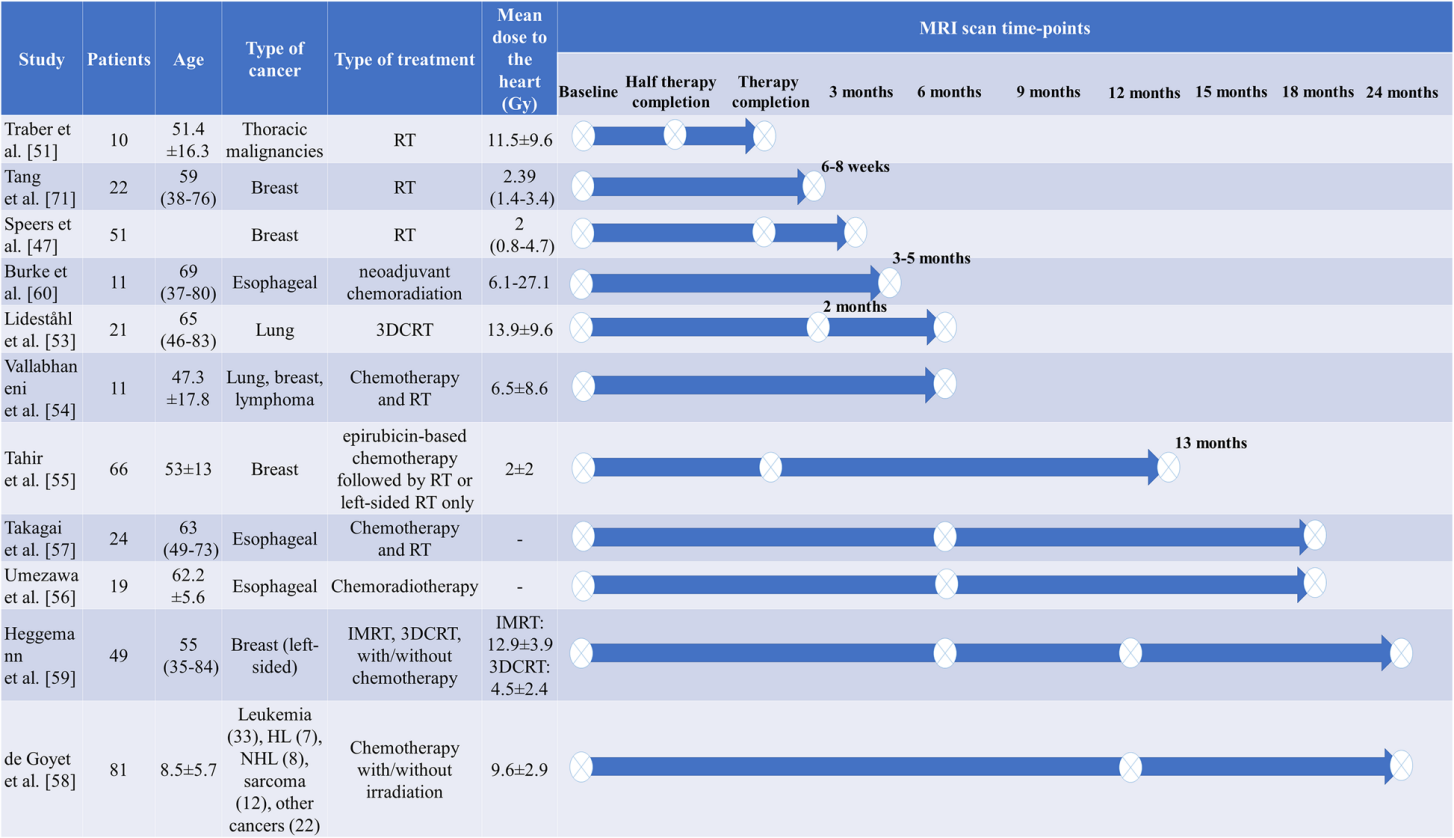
Details of longitudinal studies including number of patients, age, type of cancer, type of cancer treatment, radiation dose to the heart and timing of MRI measurements
